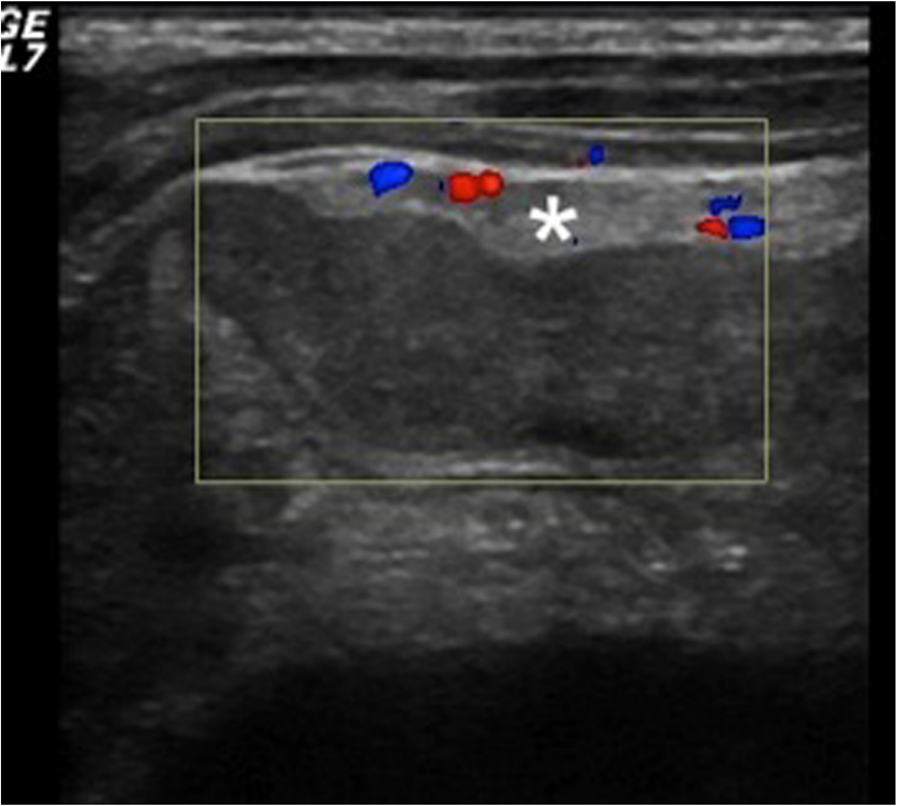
The mass was not vascularized, and surrounding mesenteric fat was hyperechoic and hypervascularized on a color Doppler ultrasound (*asterisk*)
